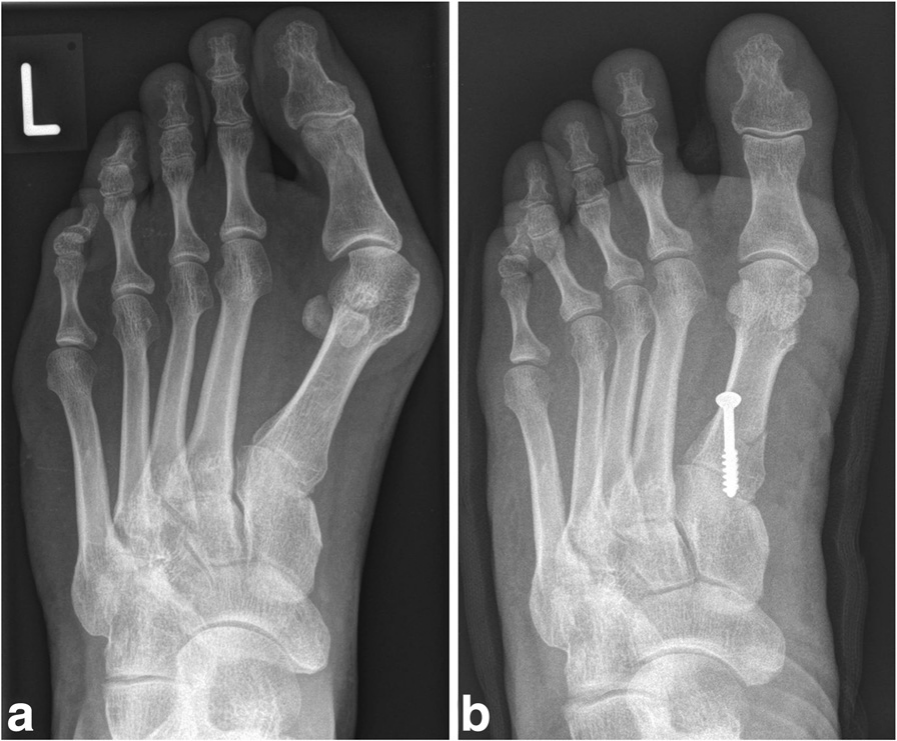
Pre- and postoperative radiographs. Weightbearing AP radiographs of the same patient presented in Figures 1, 2, 3: preoperatively (a) and two days postoperatively (b) showing a well aligned first toe without shortening of the first metatarsal.
